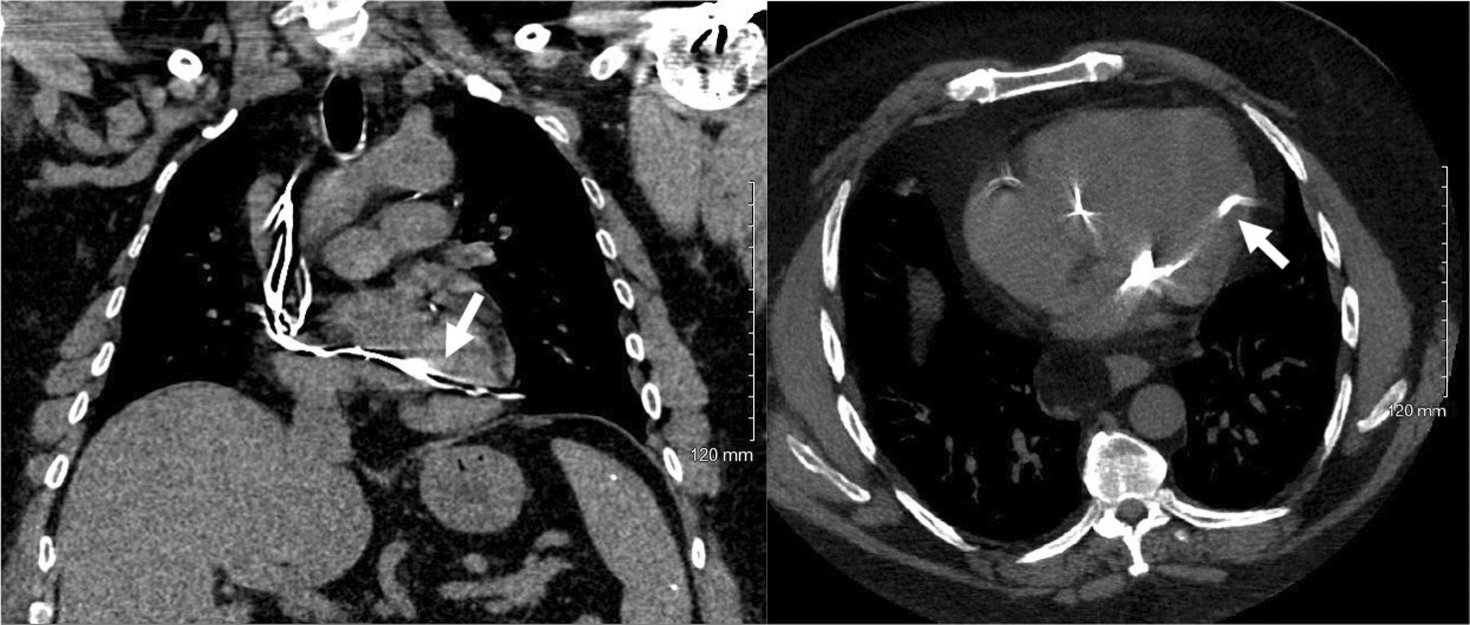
Chest CT without contrast in the coronal (left) and axial (right) plane. Despite no remark on it, the pacemaker lead is seen crossing into the left ventricle (arrows).
